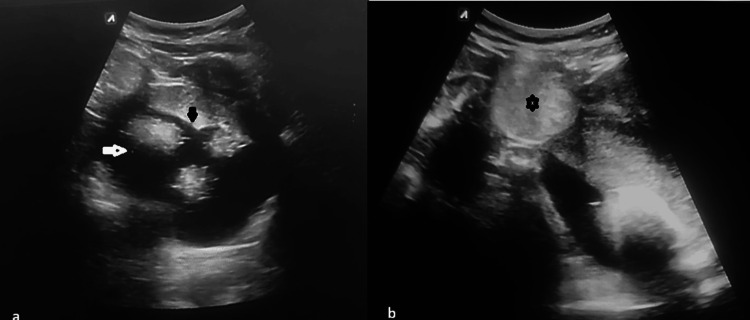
Ultrasonography images (a) Ultrasonography of the largest left ovarian cystic lesion demonstrating hyperechoic floating spherules (white arrow) and a fat-fluid level (black arrow). (b) Ultrasonography of a small right ovarian lesion demonstrating diffuse hyperechogenicity (asterisk)
